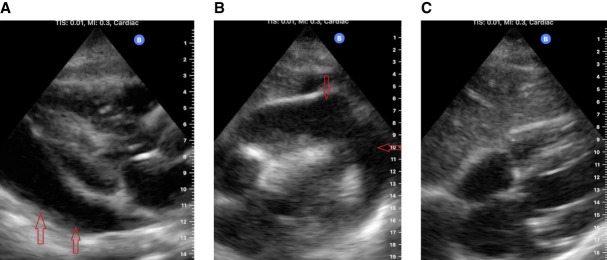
Ultrasound revealed a large circumferential pericardial effusion causing right ventricular diastolic collapse. (A) Parasternal long axis view. Red arrows indicate pericardial effusion. (B) Subcostal view. Red arrows indicate pericardial effusion. (C) Subcostal view showing resolution of pericardial effusion. LA, left atrium; LV, left ventricle; MV, mitral valve; RA, right atrium; RV, right ventricle.
